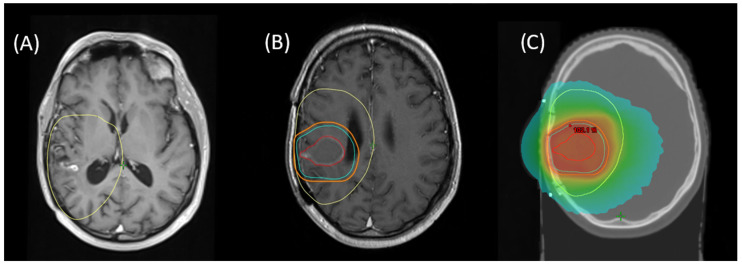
Example for (A) MRI of recurrent blood–brain barrier disruption of the right temporal lobe as a manifestation of recurrent glioblastoma, fused with initial RT planning CT to show first-line treatment PTV (yellow). (B) Planning MRI after surgical resection for fusion with planning CT with resulting target volumes for re-RT (GTV = red, CTV = cyan, PTV = orange). (C) Resulting cumulative dose distribution for re-irradiation with 39.6 Gy via helical therapy.
